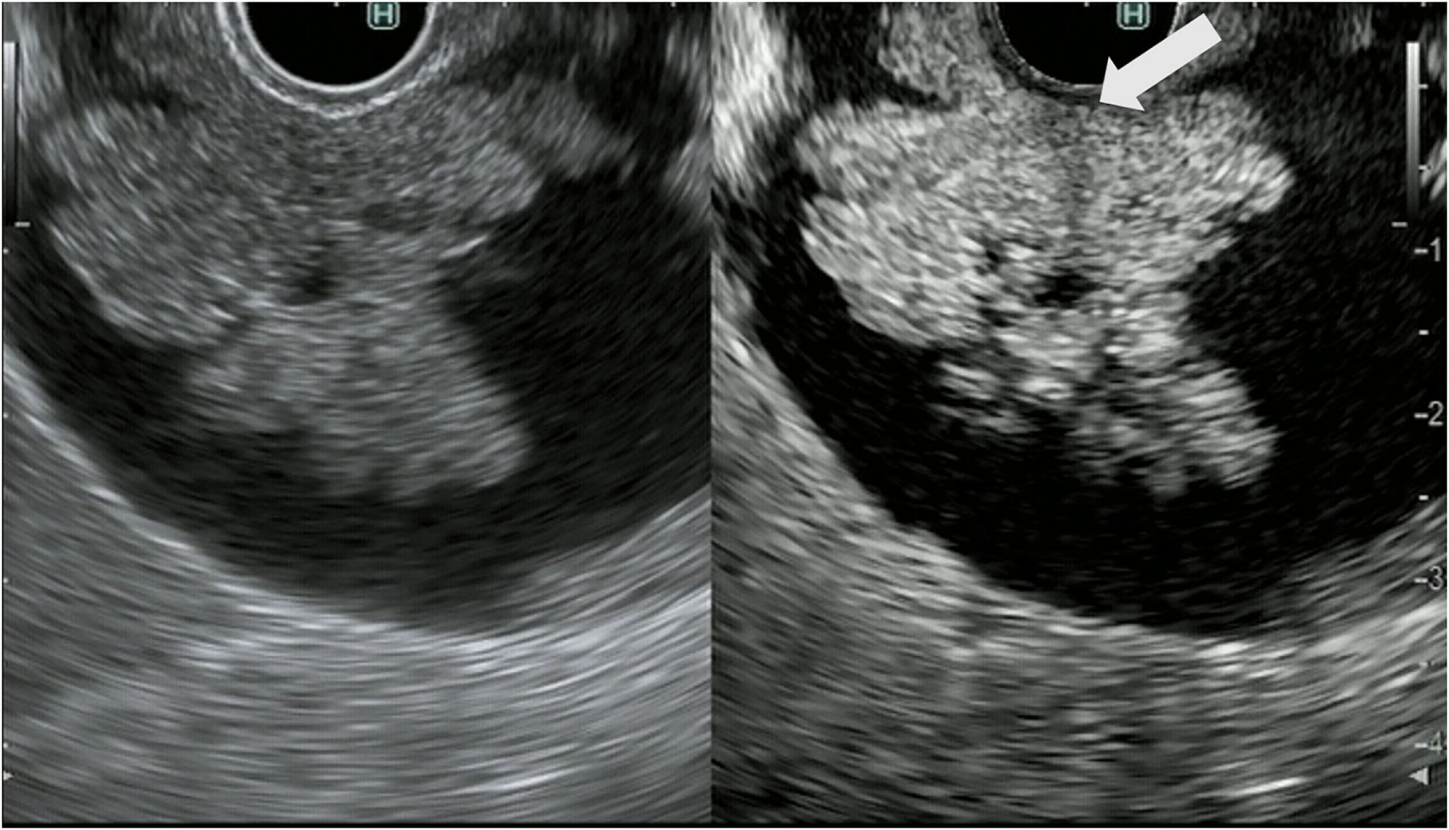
Contrast-enhanced harmonic endoscopic ultrasound (EUS) for gallbladder polypoid lesions. Gallbladder adenocarcinoma: The polypoid lesion has a perfusion defect area (arrow).
